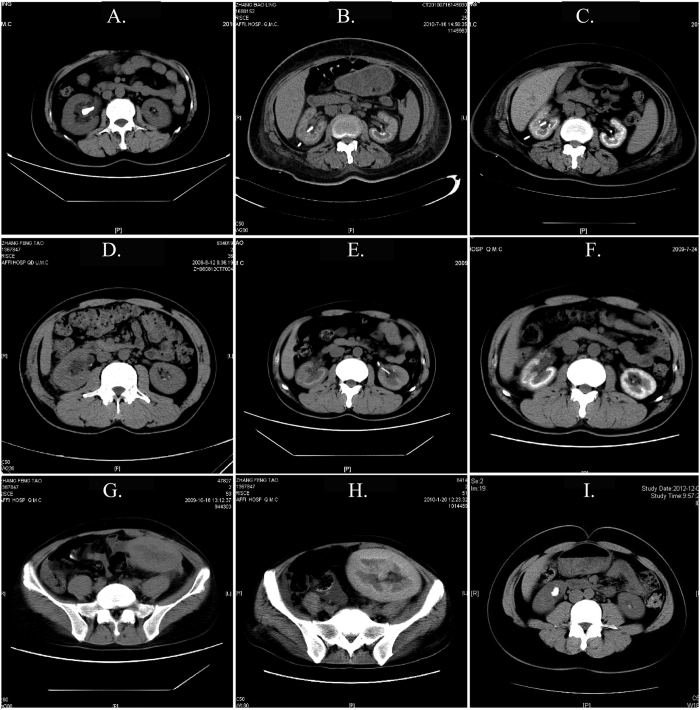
CT scanning at different stages of three Chinese siblings of IIa, IIe and IIf with Primary Hyperoxaluria Type 1. (A–C) CT scanning of bilateral kidneys of proband IIa; (D–H) CT scanning of bilateral kidneys of sibling IIe; (I) CT scanning of bilateral kidneys of sibling IIf.
